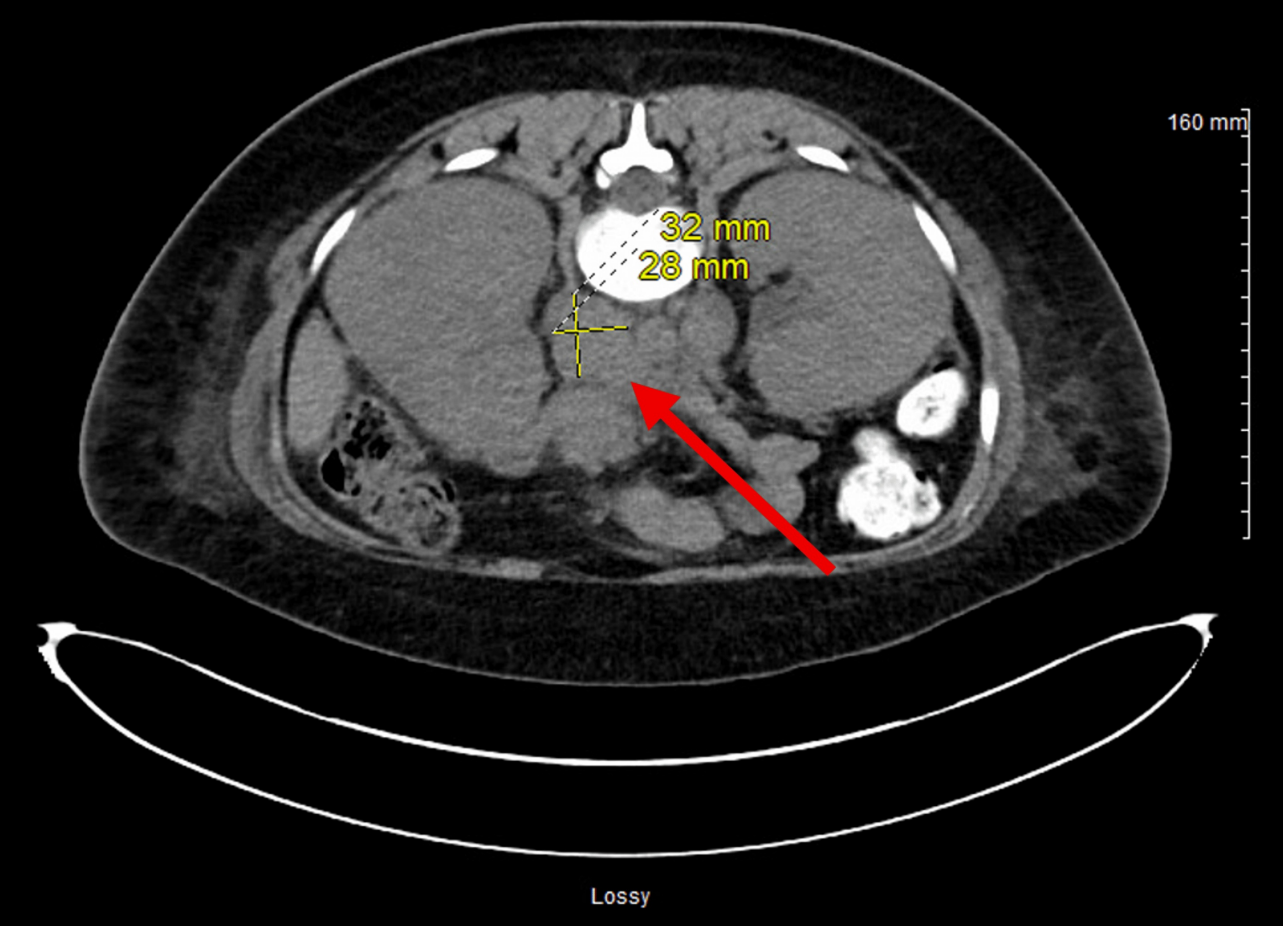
CT-guided biopsy of retroperitoneal lymphadenopathy CT-guided biopsy was performed in a 29-year-old female with abnormal bilateral renal appearance and interval development of retroperitoneal lymphadenopathy. Pre-procedure imaging demonstrated a 3.2 × 2.8 cm conglomerate of lymph nodes in the right retroperitoneum. The image shows successful needle placement targeting the retroperitoneal lymph node conglomerate for tissue sampling (red arrow).
